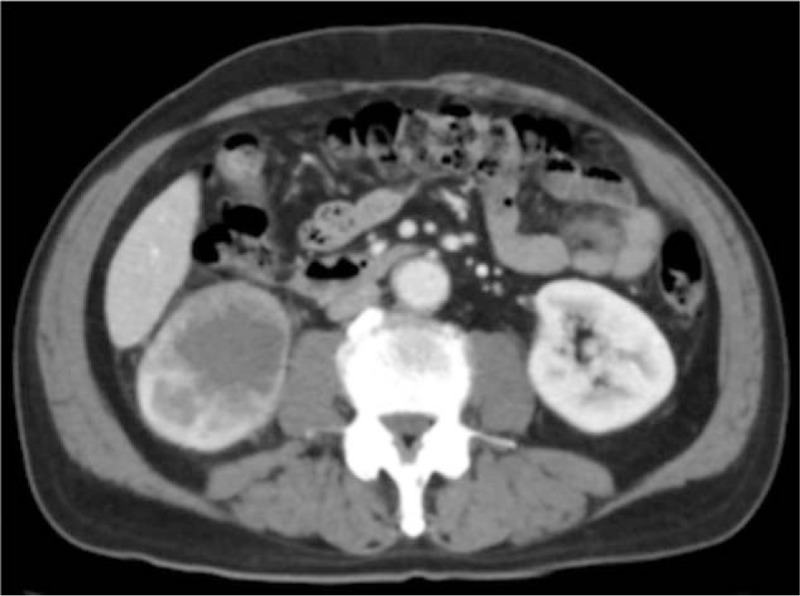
Contrast-enhanced abdominal CT revealed a poorly enhanced tumor of 55 mm in diameter in the inferior pole of the right kidney. CT = computed tomography.
